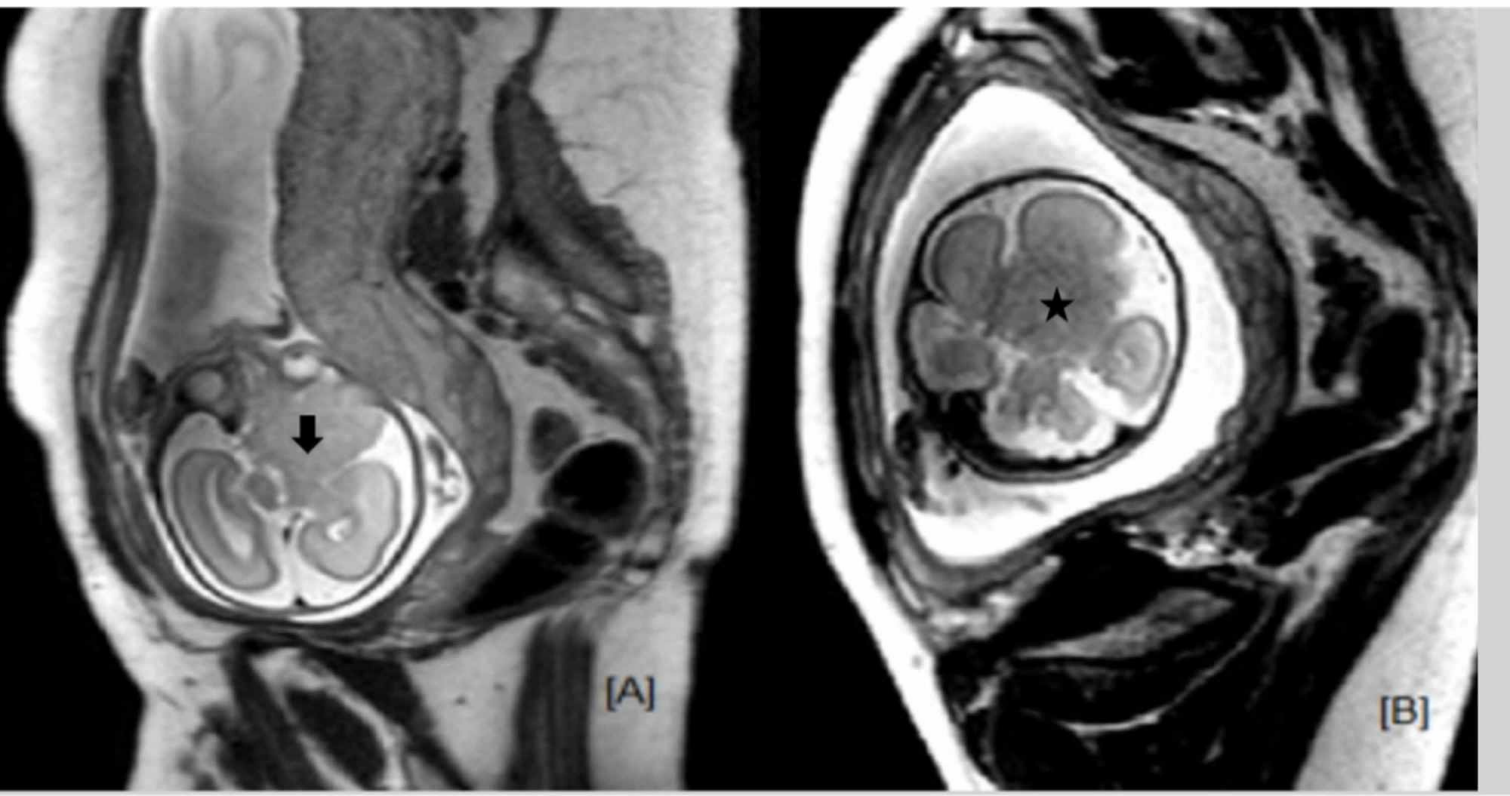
Fetal MRI brain. (A) Axial T2WI HASTE image showing ill-defined round mass (arrow) in left frontal lobe. (B) Coronal image showing inferior extent of the lesion into the thalami (star). HASTE: Half-Fourier acquired single shot turbo spin echo
